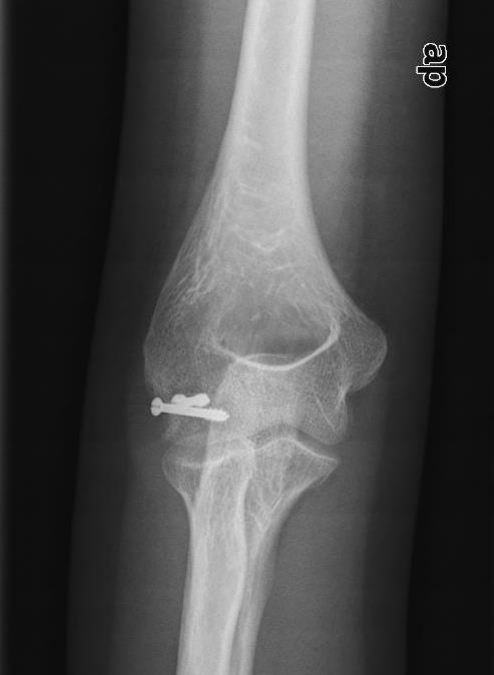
Anteroposterior radiograph one year after the surgery, showing the fracture consolidation.
